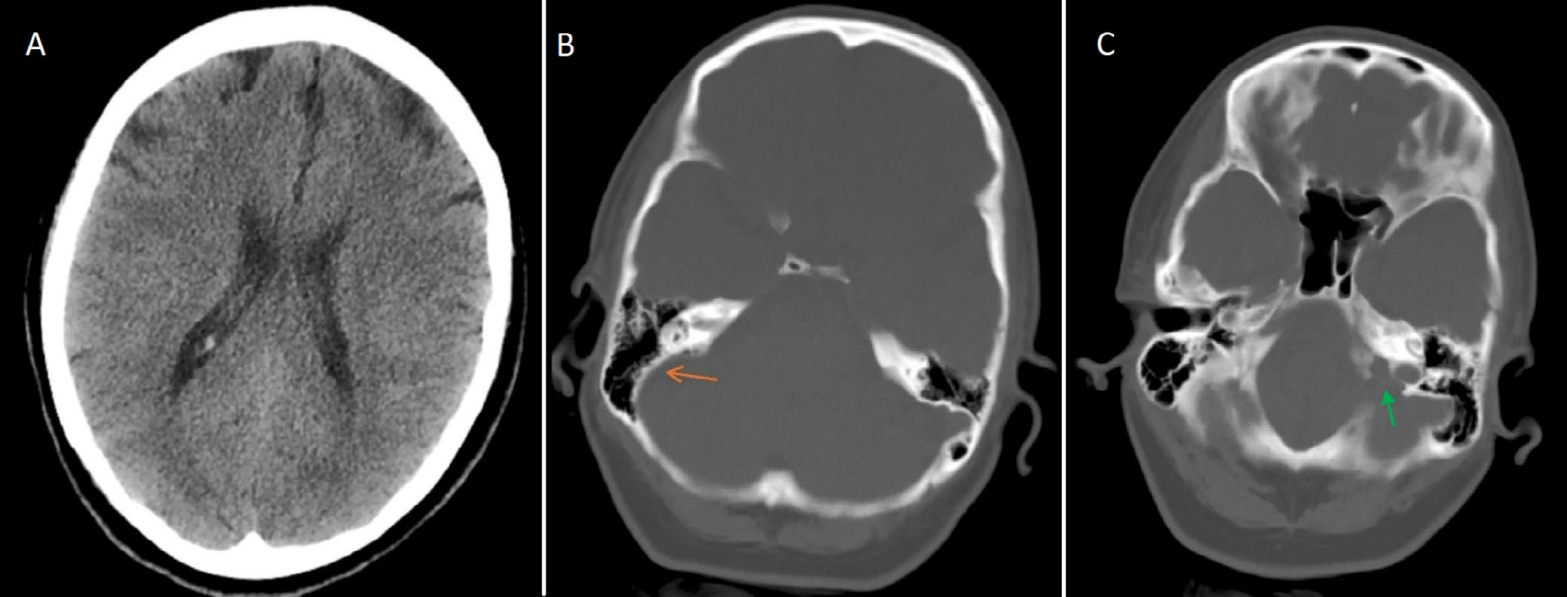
Brain computed tomography (CT). (A) soft tissue window, (B) and (C) bone window. No signs of intracranial hemorrhage or ventriculomegaly. The orange flash in the right image shows a lack of a sigmoid notch on the right side. The green arrow indicates the left jugular canal, which is absent on the right side.
